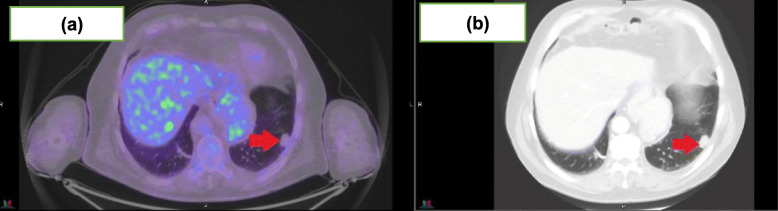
73 year old man with imaging for metastatic cutaneous squamous cell carcinoma to the lung post 12 months of cemiplimab 350 mg/3-weekly. a demonstrating a CMR to treatment and (b) demonstrating ongoing RECIST1.1 PR with measurable disease on CT
